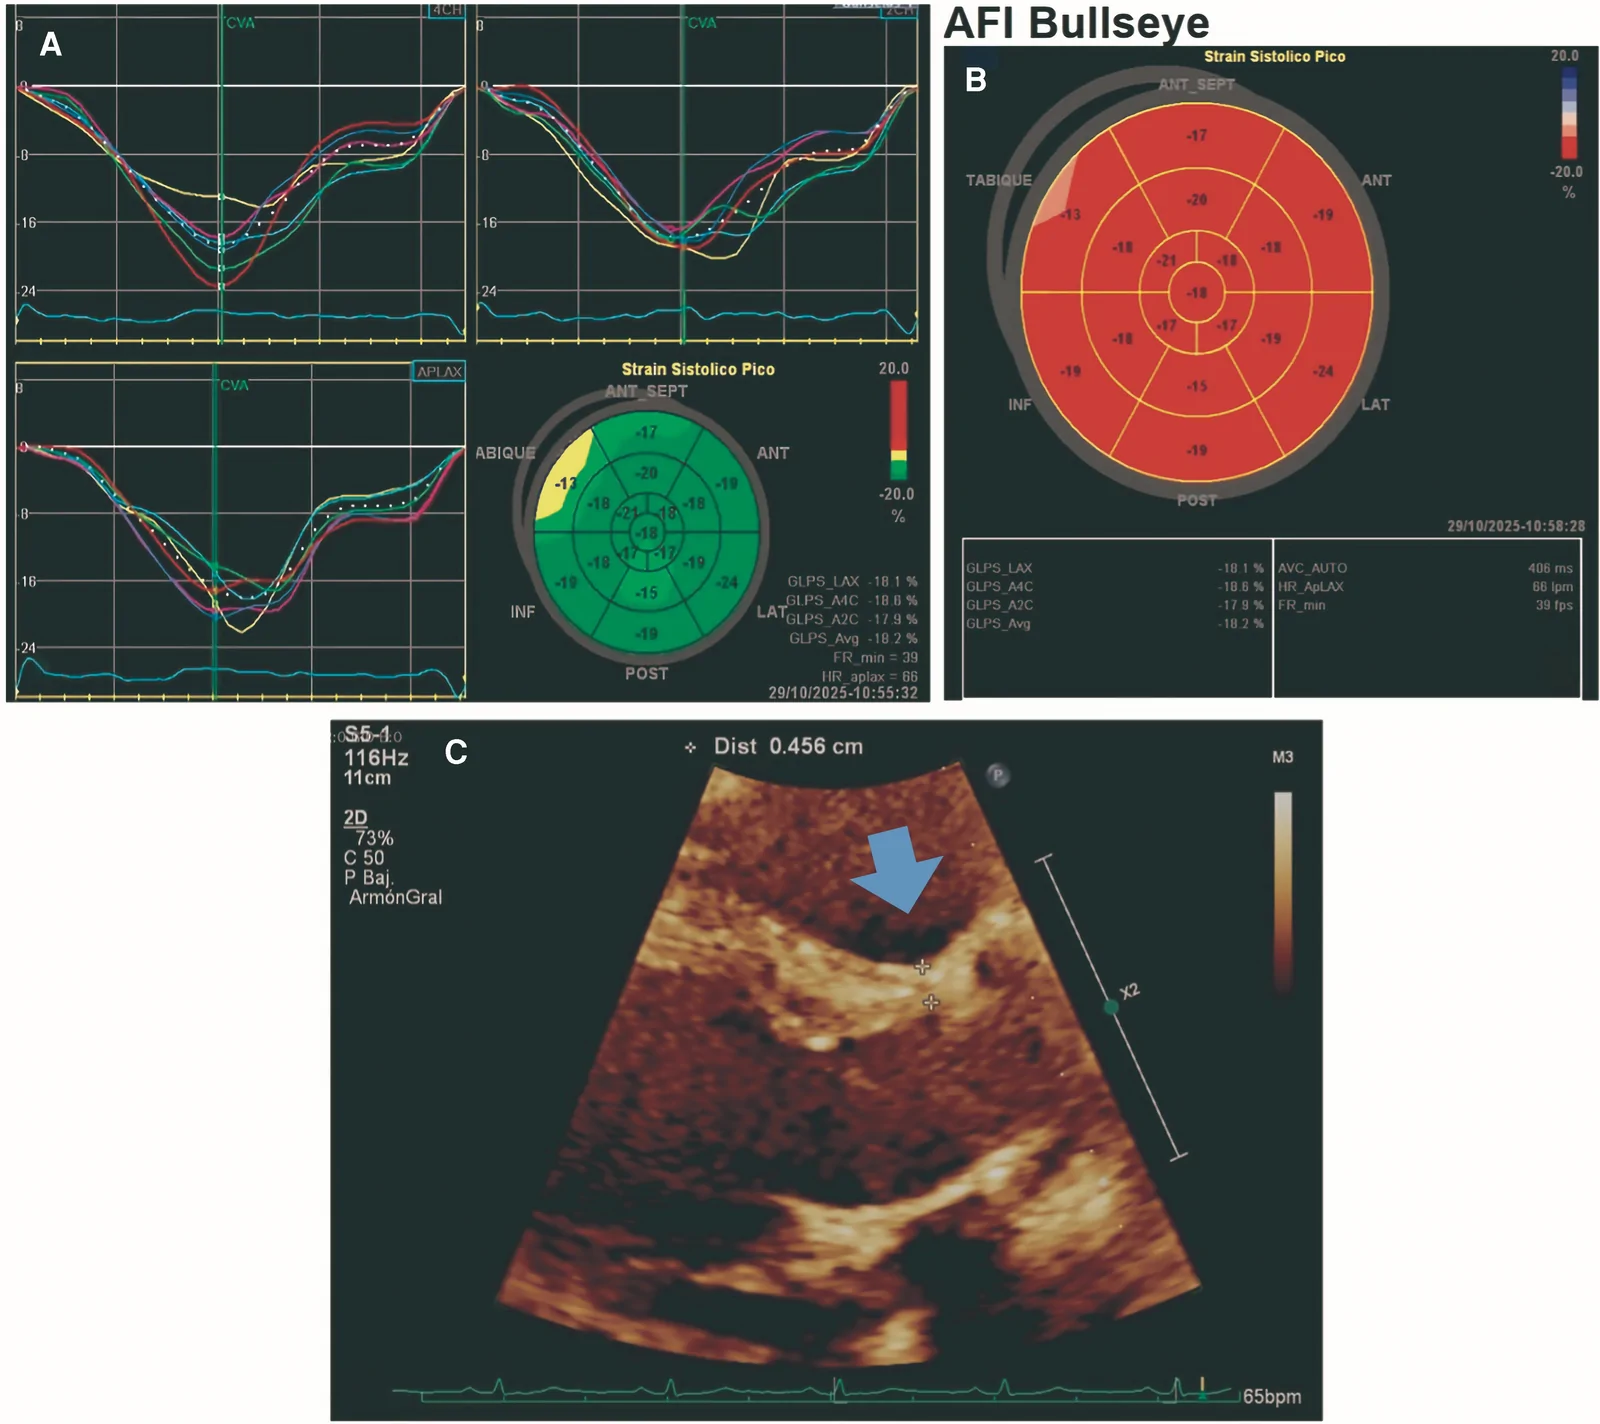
Speckle-tracking echocardiography. *(A)* Longitudinal strain curves showing a global longitudinal strain of −18%, consistent with subclinical to mild left ventricular dysfunction and a regional pattern suggestive of patchy myocardial involvement. *(B)* Polar map (‘bull’s-eye’) demonstrating regional reduction of longitudinal strain, predominantly involving the basal and mid-septal segments. *(C)* Basal thinning of the interventricular septum is also evident (arrow).
